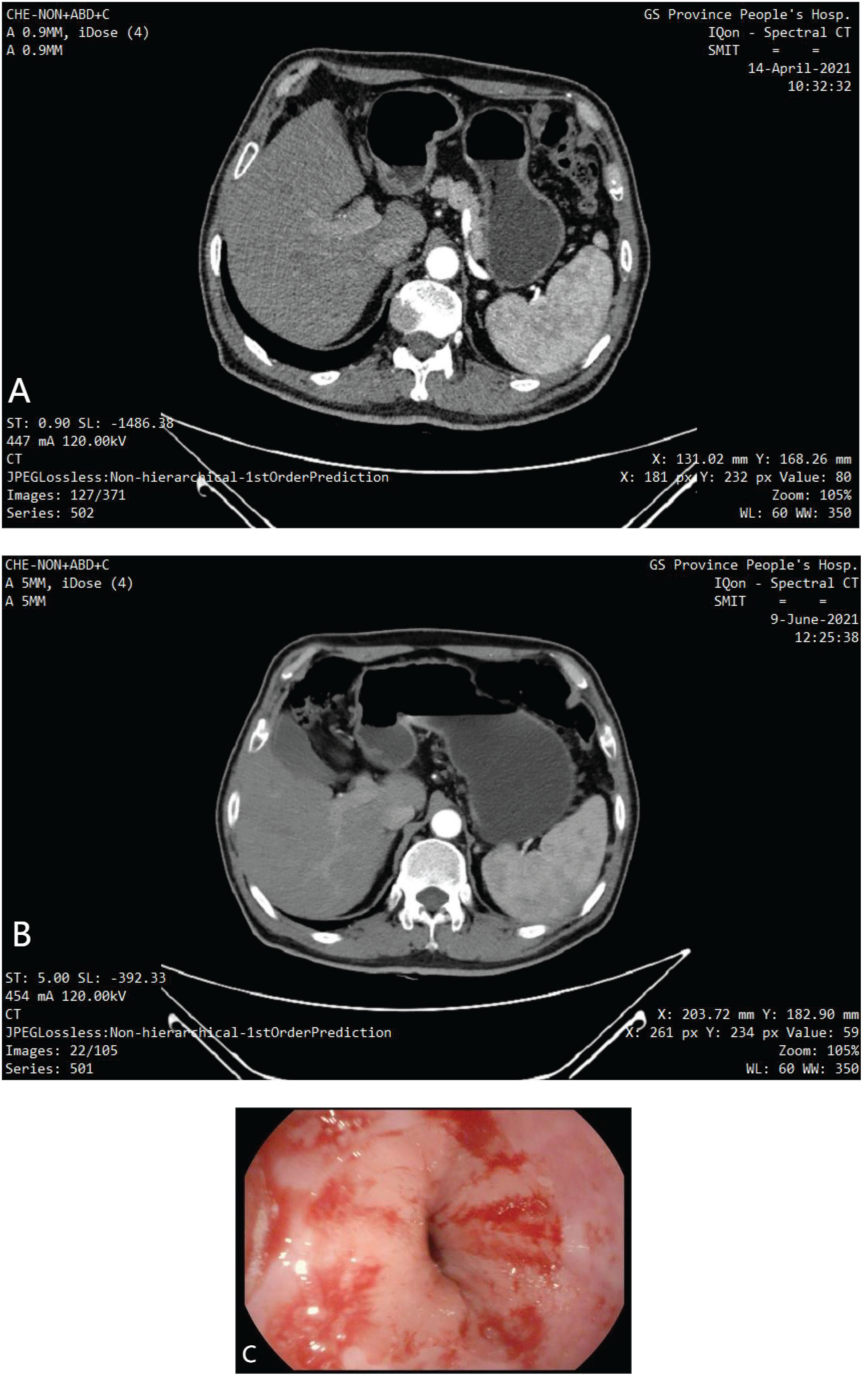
(A) Enhanced CT: The gastric wall was thickened and mildly enhanced at the gastric angle and the side of the lesser curvature of the gastric sinus, with a thicker area of about 12 mm, the plasma membrane surface was still smooth, and no obvious enlarged lymph node shadow was seen around. Considered GC (T3N0M0). (B) Enhanced CT: The gastric lumen is well filled, no restrictive thickening of the gastric wall, and no abnormal enhancement of the gastric wall in the sinus region. Please combine with the clinical treatment history. (C) Electrogastroscopy: The mucosal folds of the gastric body were neatly arranged, and multiple patches of congested erosions were seen.
